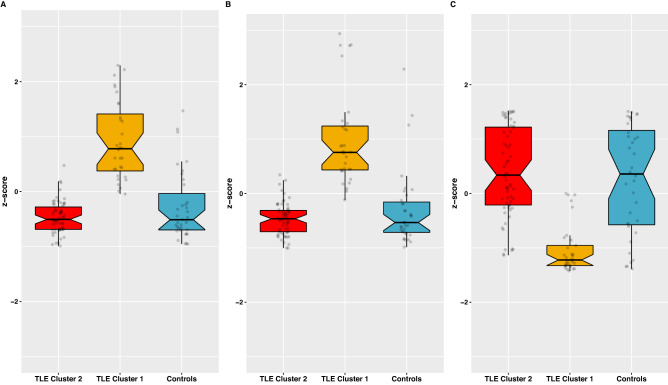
(A) Local efficiency, (B) Global efficiency, and (C) modularity index across ML-derived TLE clusters (red and yellow) and healthy controls (blue) for morphological MRI.
